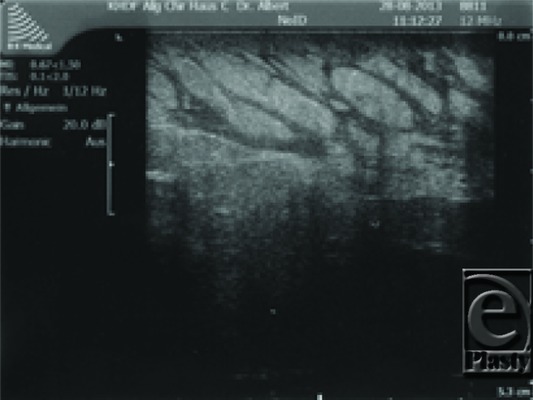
Postoperative sonographic control with large lymphatics but without pseudocysts.
